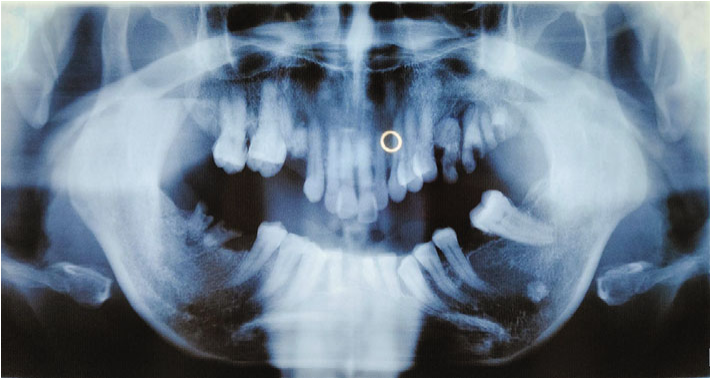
An orthopantomogram revealed slight alveolar bone resorption around the interdental Teeth 11, 12, 21 and 22, as well as multiple Dental Root Teeth 15, 26, 27, 28 and 47.
